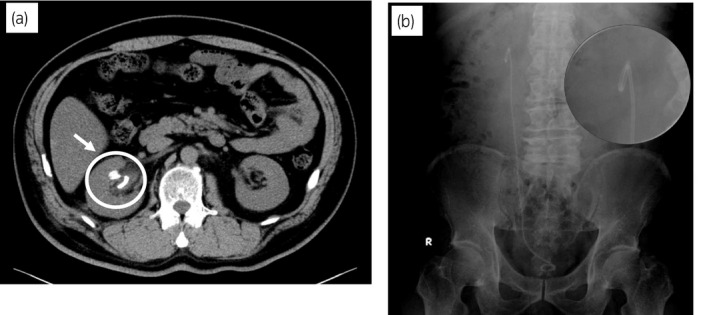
(a) Plain CT showing residual stone. (b) The preoperative imaging that X‐ray KUB did not show encrusted ureteral stent.
